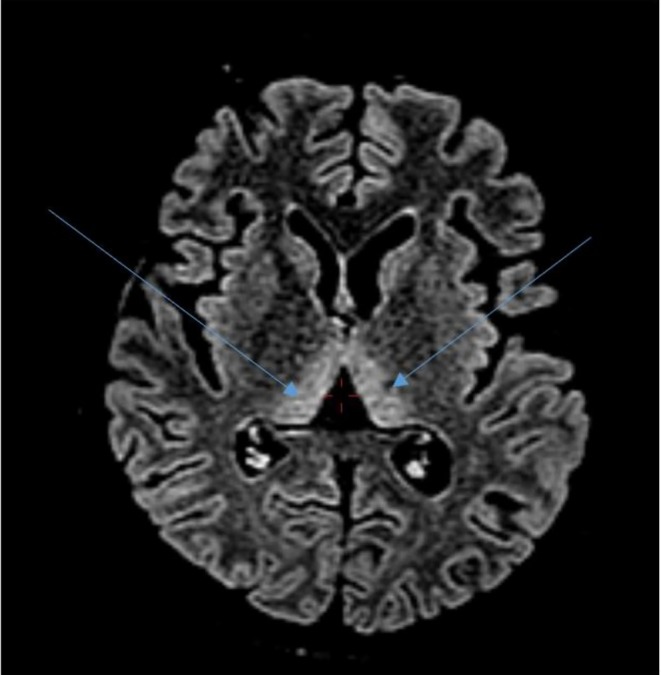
MRI of the brain (T2 sequence) showing high intensity signal in bilateral medial thalami.
